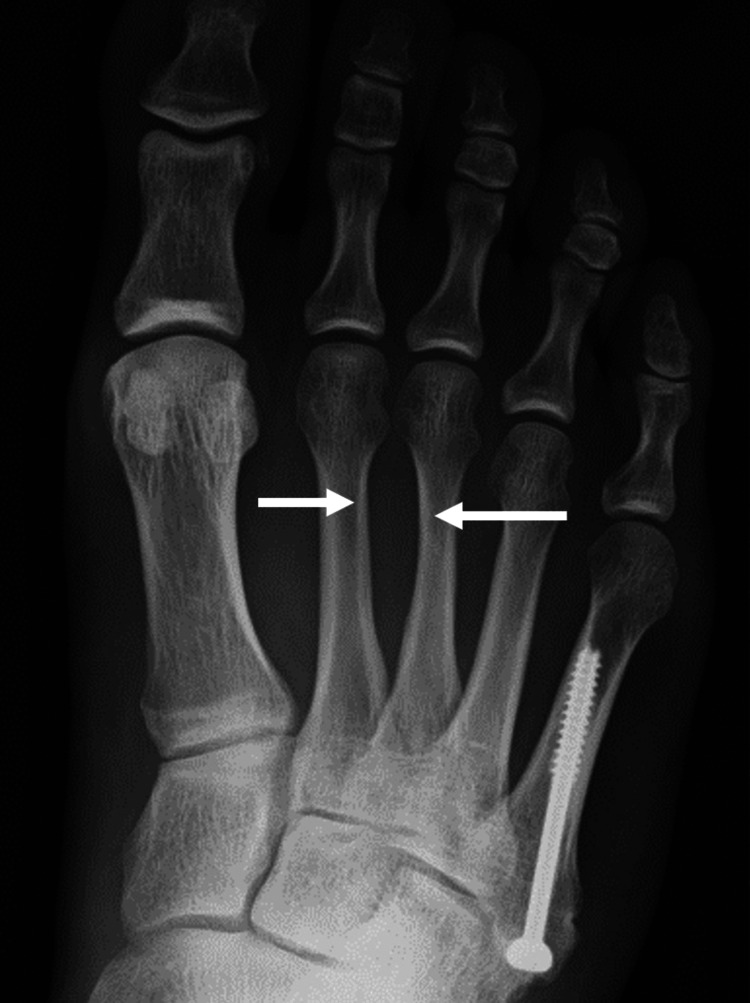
Plain radiograph showing cortical bone thinning in the right second and third metatarsals (white arrow).
